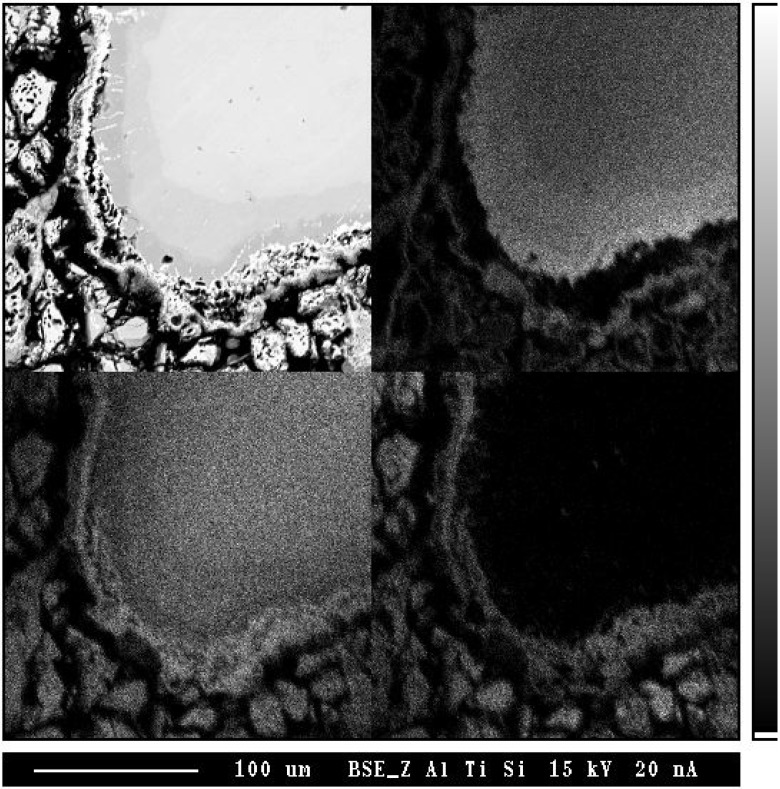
The BSE image (a) and X-ray maps (with respect to (b) Al, (c) Ti and (d) Si) of TiAl/TiSi_2_ powder mixture annealed at 1,100 °C for 40 h [7].
